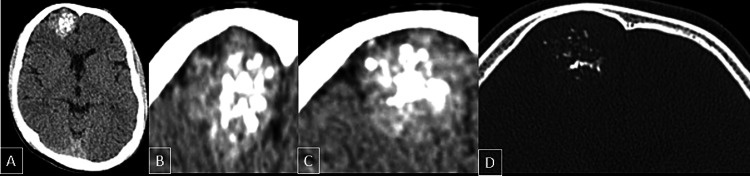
Non-contrast CT scan. The scan showed a cortical lesion in the anterior right frontal lobe in the right parasagittal plane with multiple hyperdense foci within (A-C), representing calcifications/low-grade hemorrhage.  Bone window reconstruction reveals bone scalloping and thinning of the right frontal bone overlying the lesion (D).
